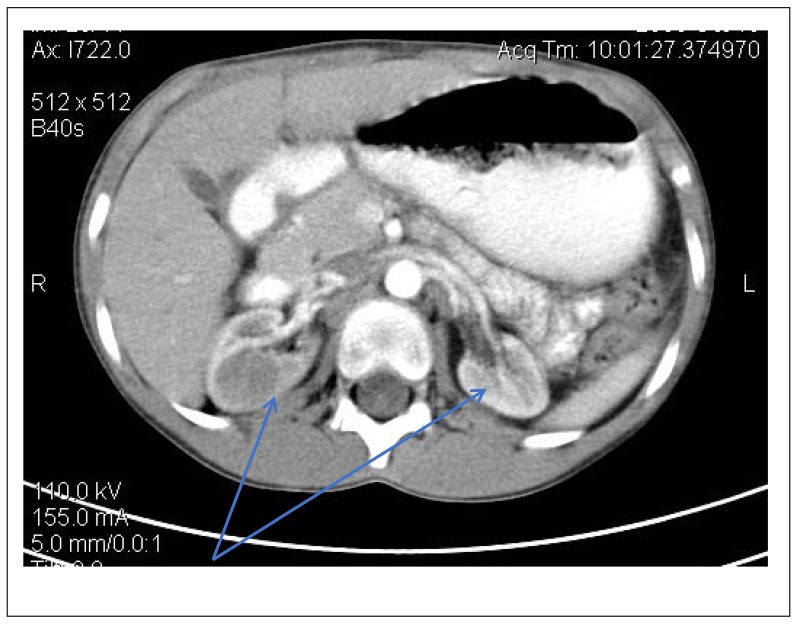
Abdominal CT scan—expansive renal formations replacing renal parenchyma and with a mass effect (blue arrow).
